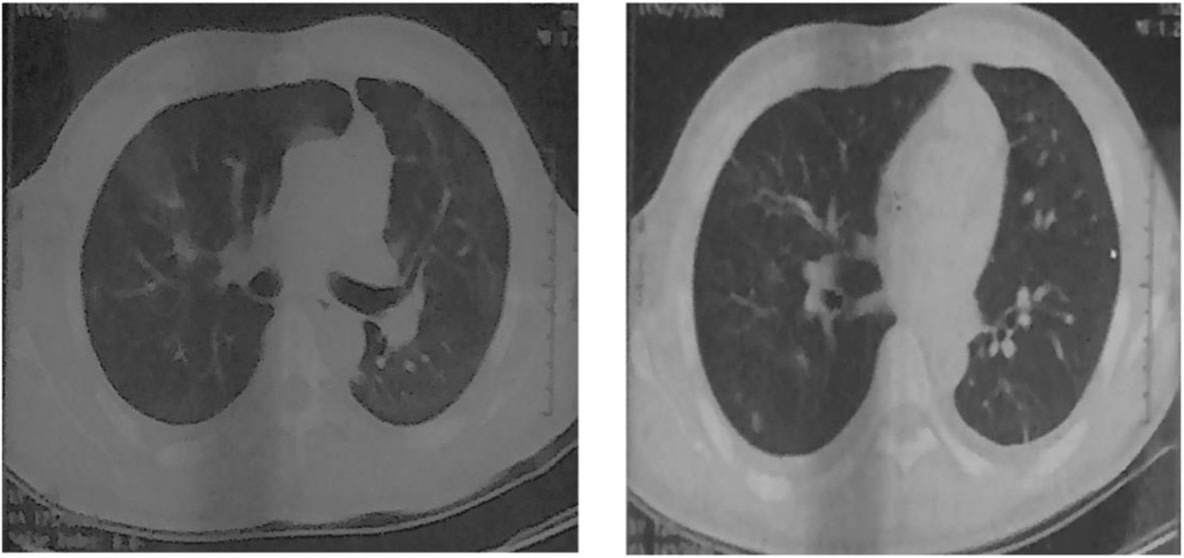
Repeated chest CT revealing infiltration markedly improved and fibrous proliferation, calcification
